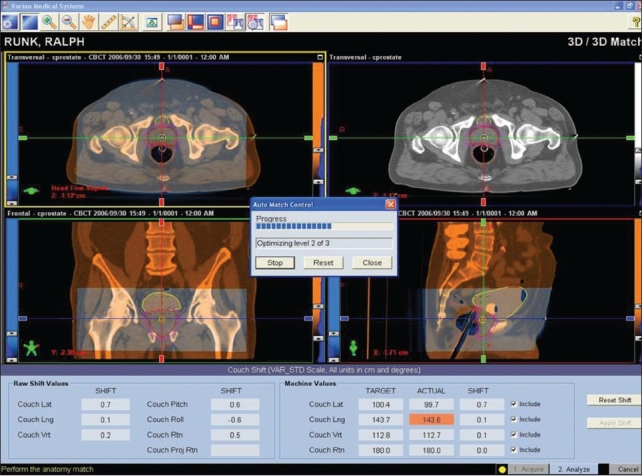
Auto-matching for positioning of a patient based on volumetric CBCT image taken prior to treatment
